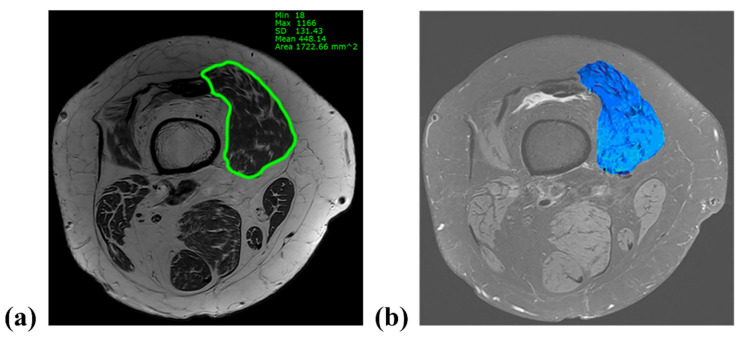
Measurement of cross-sectional area (CSA) and fat infiltration (FI) ratio on axial magnetic resonance imaging. (a) The vastus medialis (VM) CSA was acquired from the axial proton density image after marking the VM margin using the embedded tools of the picture archiving communication system software. (b) The area corresponding to the VM was color-mapped with 16 different colors for each signal. Color pixels derived from the fat signal were extracted, and the FI ratio was defined as the percentage of the number of fat pixels to the sum of the VM pixels.
